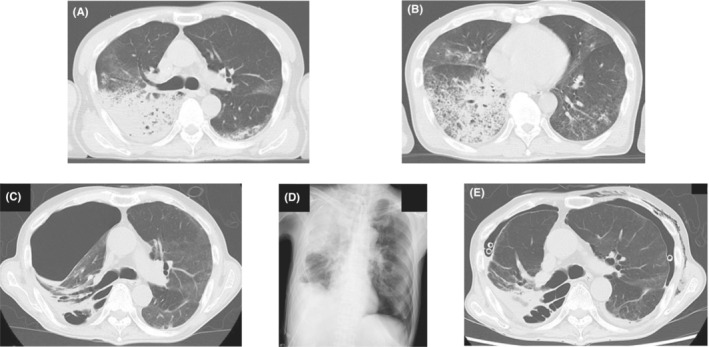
(A, B) Contrast computed tomography on admission shows infiltration shadows on both sides. (C) Computed tomography shows second right‐sided pneumothorax. (D) Chest X‐ray shows first left‐sided pneumothorax. (E) Computed tomography shows bilateral pneumothorax after bilateral tube drainage.
